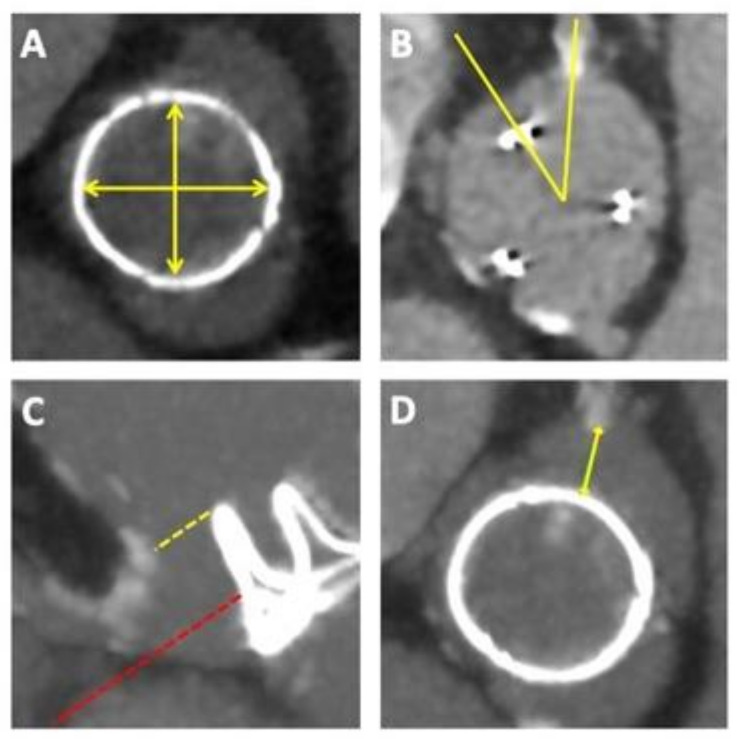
Pre-procedural planning for ViV-TAVI. Pre-procedural computed tomography is required to evaluate the (A) true internal dimensions of the implanted SAV, (B) the alignment of the SAV with respects to the native coronaries, (C) the coronary and sinus heights in relation to the top of the SAV frame, or if no posts are visible, then anticipated heights of leaflets and (D) the gap between the frame and/or leaflets and coronary ostia to estimate the risk for coronary obstruction.
